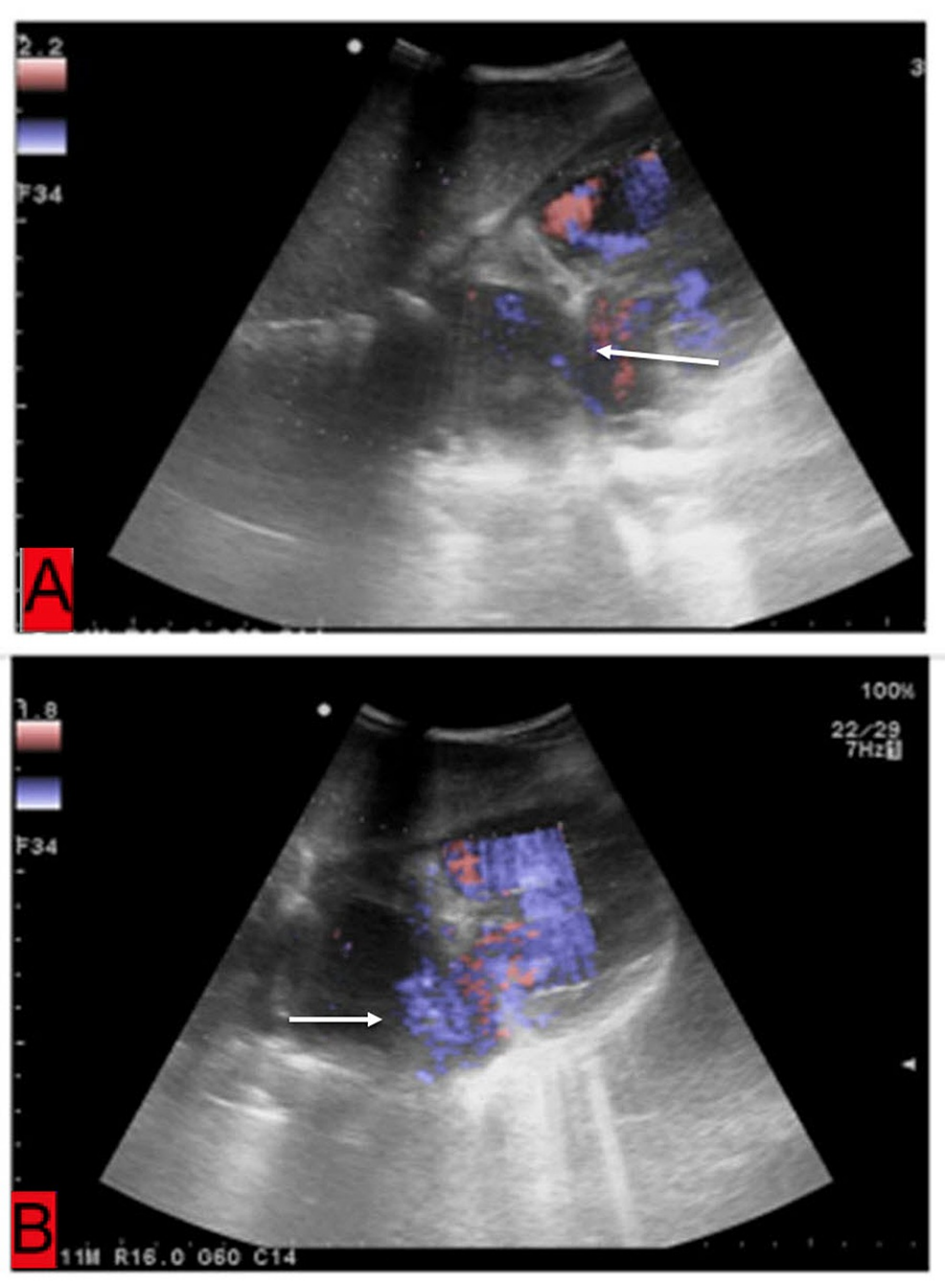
(A and B) Gray-scale sonographic visualization of heart of a five-year-old child suffering from heterotaxy syndrome revealing a large defect in the inter-atrial septum.
